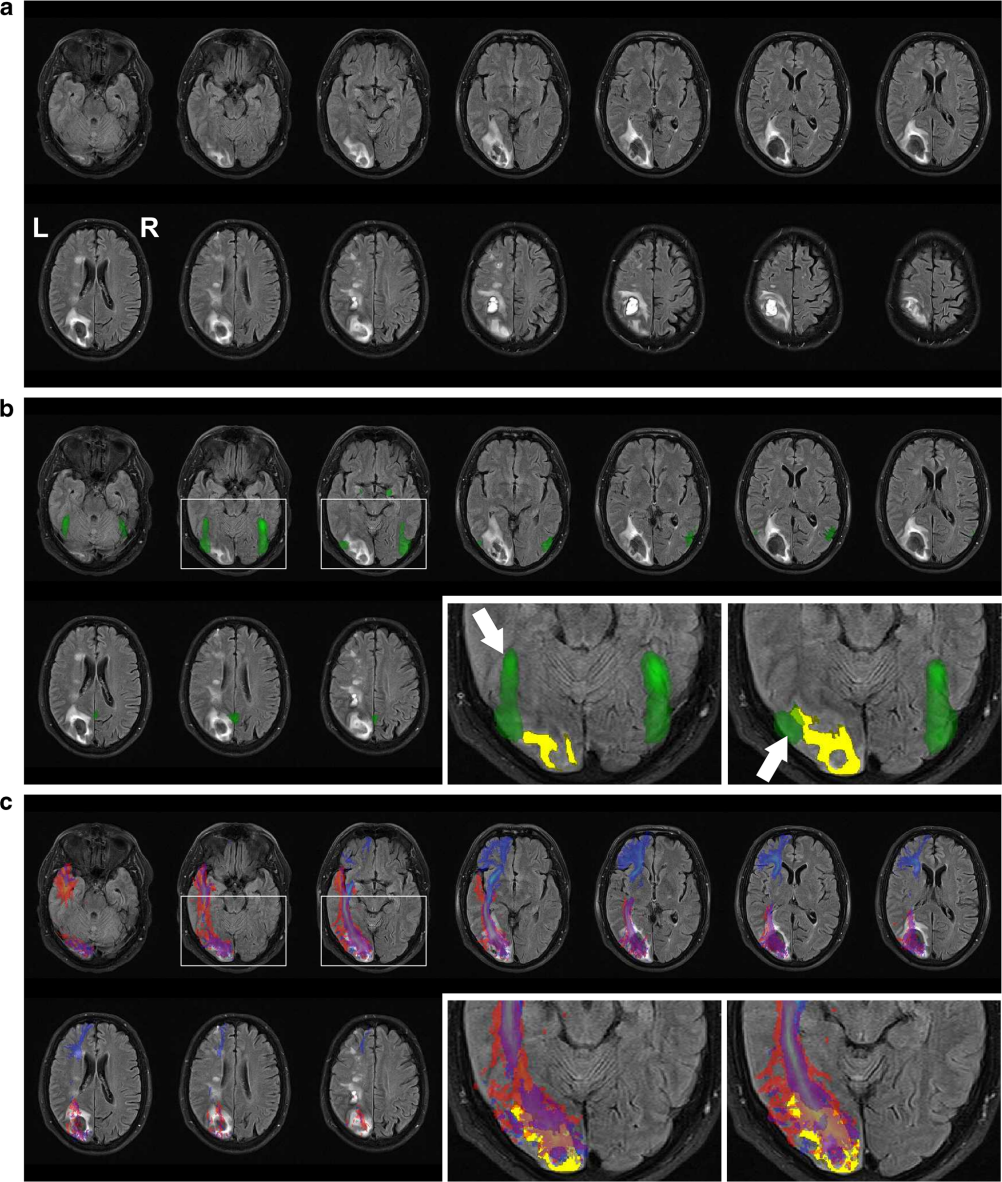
Patient’s brain MRI. a Fluid-attenuated inversion recovery (FLAIR) image of the patient’s brain, with transaxial slices (in neurological convention) revealing the presence of two left-hemispheric lesions, one centered around the inferior parietal lobule and the other one in the occipito-temporal territory. b Superimposed on the FLAIR image (green color) are the functional MRI probability maps of the “Atlas of Social Agent Perception” [44], representing the activation likelihood in a large cohort of the healthy population for the processing of face images. The maps have been warped to the patient’s native brain space. Rectangular areas (white outlines) of two adjacent transaxial slices are shown in greater magnification in the bottom right insets, with yellow indicating lesioned brain tissue automatically segmented by a lesion growth algorithm ([45]; initial threshold determined by visual inspection) as implemented in the LST toolbox version 2.0.15 (www.statisticalmodelling.de/lst.html) for SPM (www.fil.ion.ucl.ac.uk/spm). A downward white arrow (left inset) points to the approximate position of the fusiform face area (FFA), which is most likely not affected by the lesion. In turn, the occipital face area (OFA), indicated by an upward white arrow (right inset), is likely affected by the lesion. c Superimposed on the FLAIR image are the probabilistic tractography maps of the inferior longitudinal fasciculus (red color) and of the inferior fronto-occipital fasciculus (blue color) stemming from the “JHU White matter tractography atlas” [46]. The maps, representing the white matter tract probability in the healthy population, have been warped to the patient’s native brain space. As visible in the two rectangular (white outline) magnified insets, both white matter fascicles are most likely affected by the lesion (yellow color)
